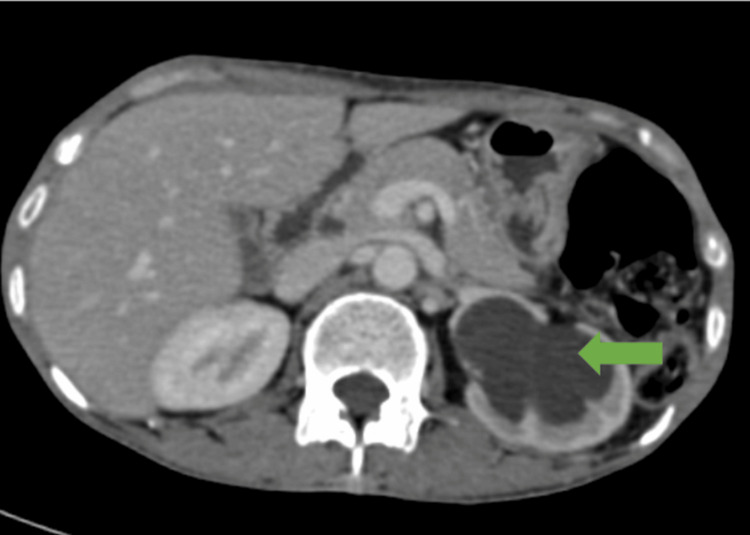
Contrast-enhanced computed tomography axial section shows dilatation of the left renal pelvis (green arrow) due to the obstruction of the left vesicoureteric junction by the mass lesion in the urinary bladder
